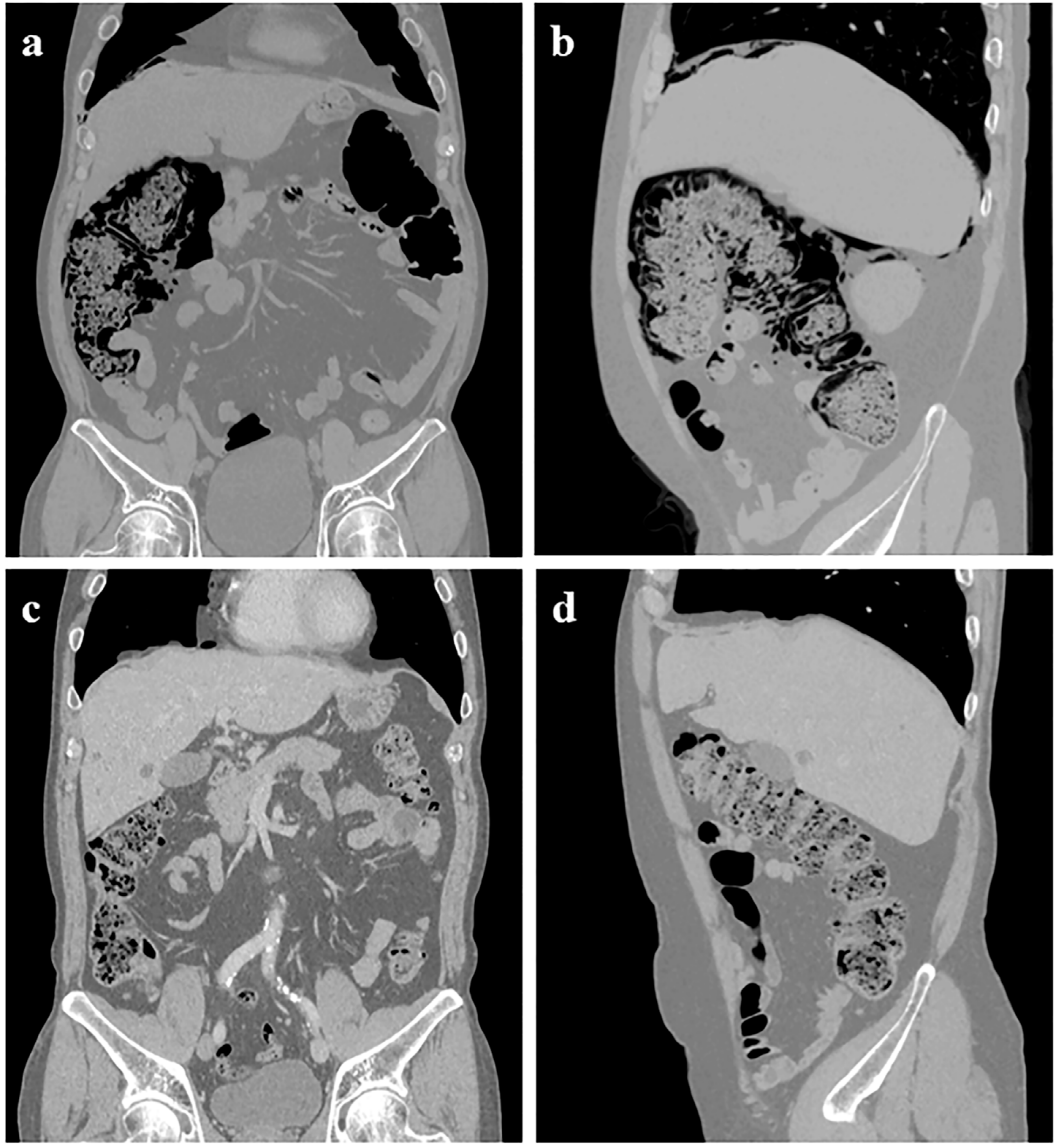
Abdominal CT showed pneumoperitoneum under the right diaphragm, extensive intramural air in the ascending colon, and hepatic flexure with massive air accumulation in the colon 6 weeks after starting high-dose methylprednisolone (A–B). However, these findings had almost completely disappeared on abdominal CT taken at the outpatient clinic (C–D).
